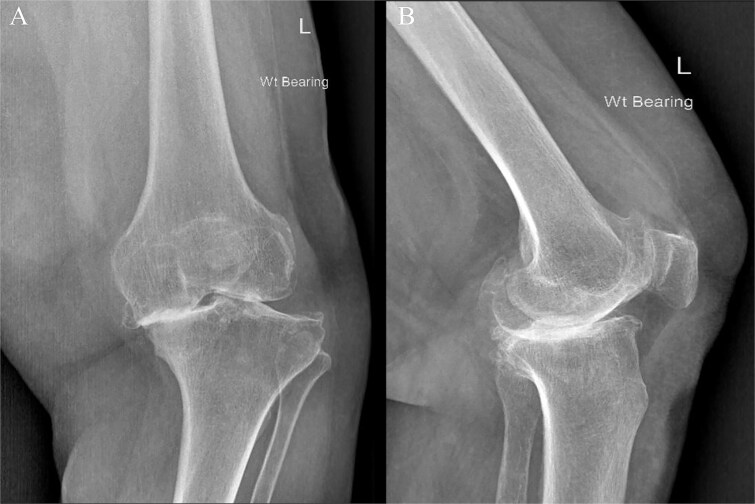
Preoperative face (A) and profile (B) X-rays showing end-stage knee arthritis with varus deformity and severe collision between the medial femoral and tibial condyles.
